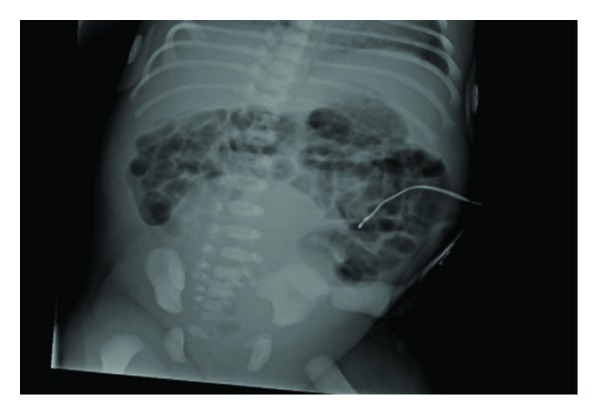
Plain abdominal radiograph of Neonate B showing infraumbilical midline abdominal opaque mass.
